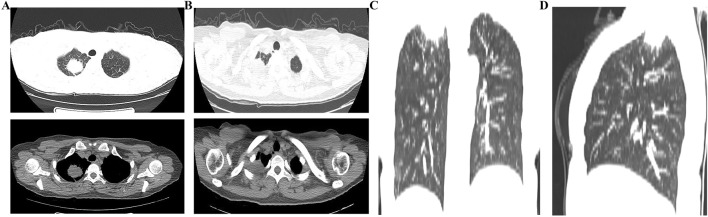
The first plain chest CT scan revealed a space-occupying lesion in the right upper lung. (A,B) Lung window and mediastinal window demonstrated a space-occupying lesion in the right upper lobe; (C,D) Coronal and sagittal plain chest CT images showed the space-occupying lesion in the right upper lobe.
